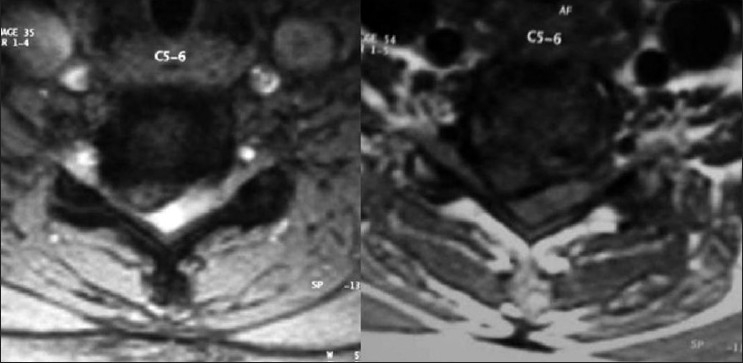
Preoperative axial T2W1 and T1W1 MRI images shows the disc herniation
